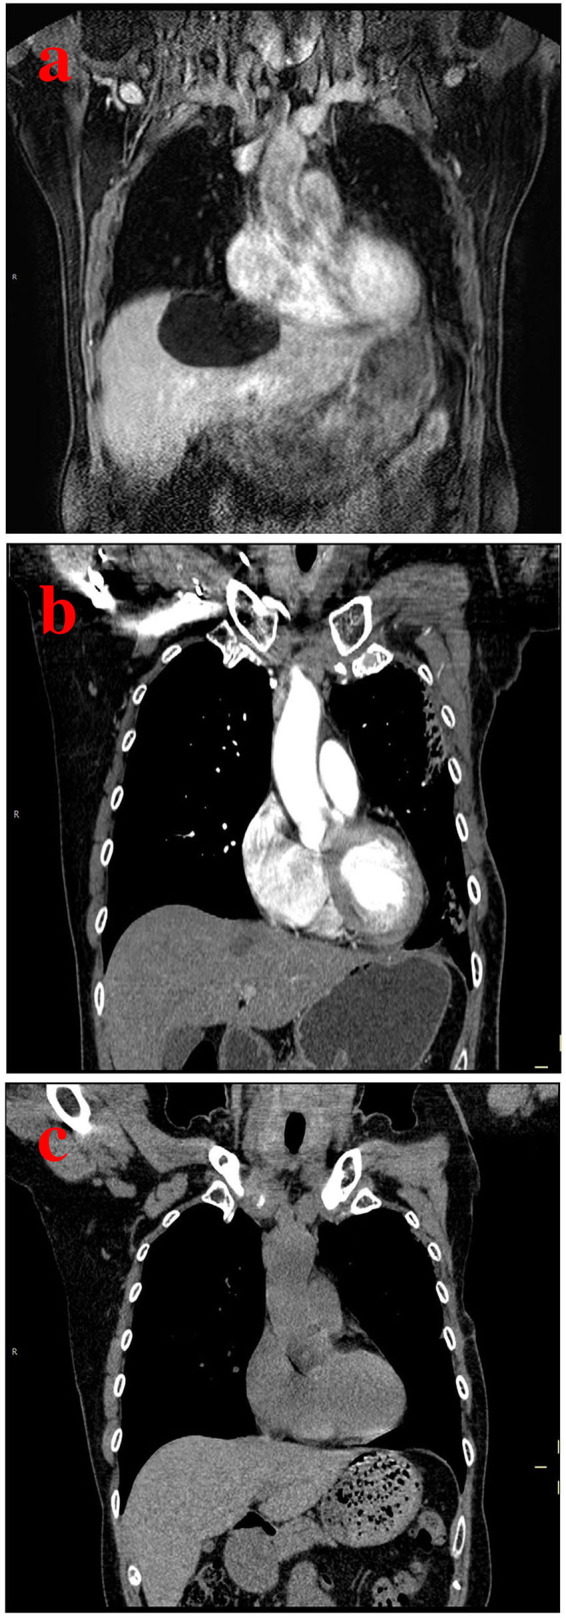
A case of a 37-year-old man with a hepatic cyst. (a) Pretreatment CT scan shows a hepatic cyst measuring 11.4 cm in length, 10.3 cm in width, and 8.9 cm in depth. (b) Follow-up CT scan after 3 months of sclerotherapy with single-session OK-432 shows a markedly decreased hepatic cyst. (c) Follow-up CT scan after 12 months shows complete regression of the hepatic cyst.
